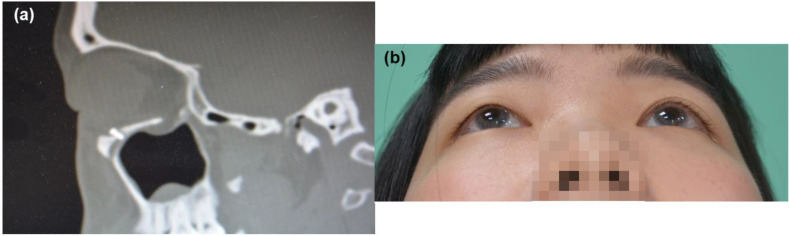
(a) Postoperative CT scan of case 1, left side orbital floor to blow out a fracture. ABCcolla® Collagen Bone graft was fixed by one screw (b) post-operation 2 months follow-up. Without any complaint was noted.
